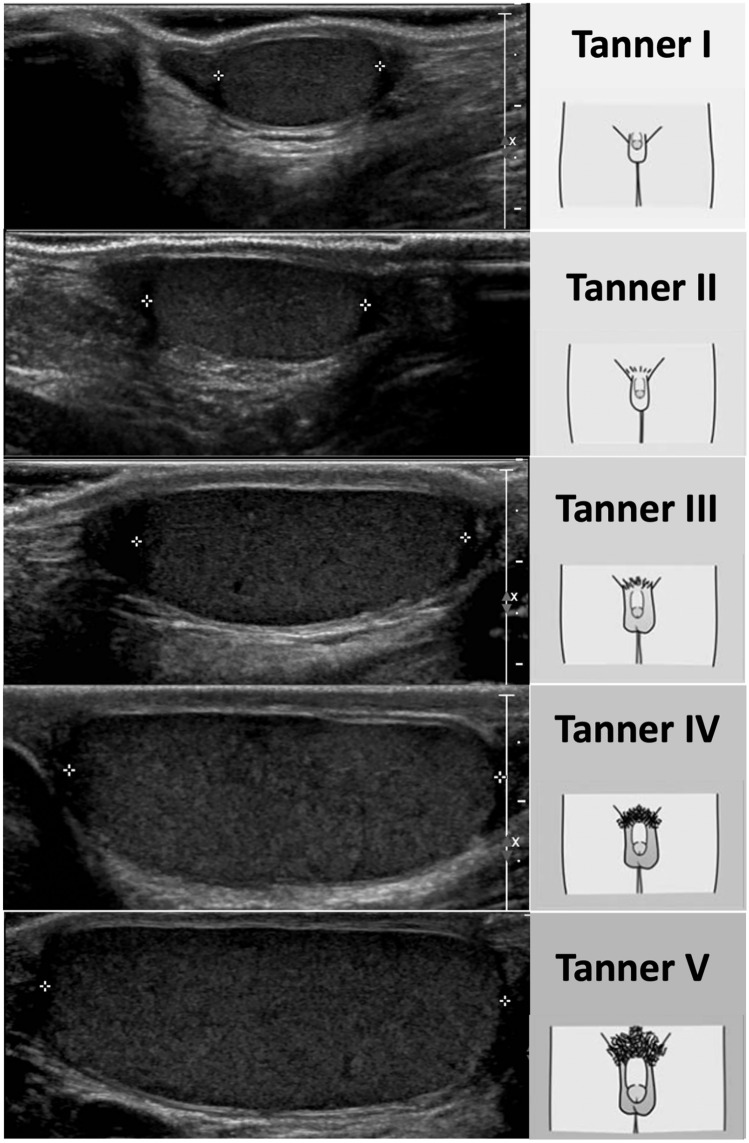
Normal testicular ultrasound according to Tanner stages
